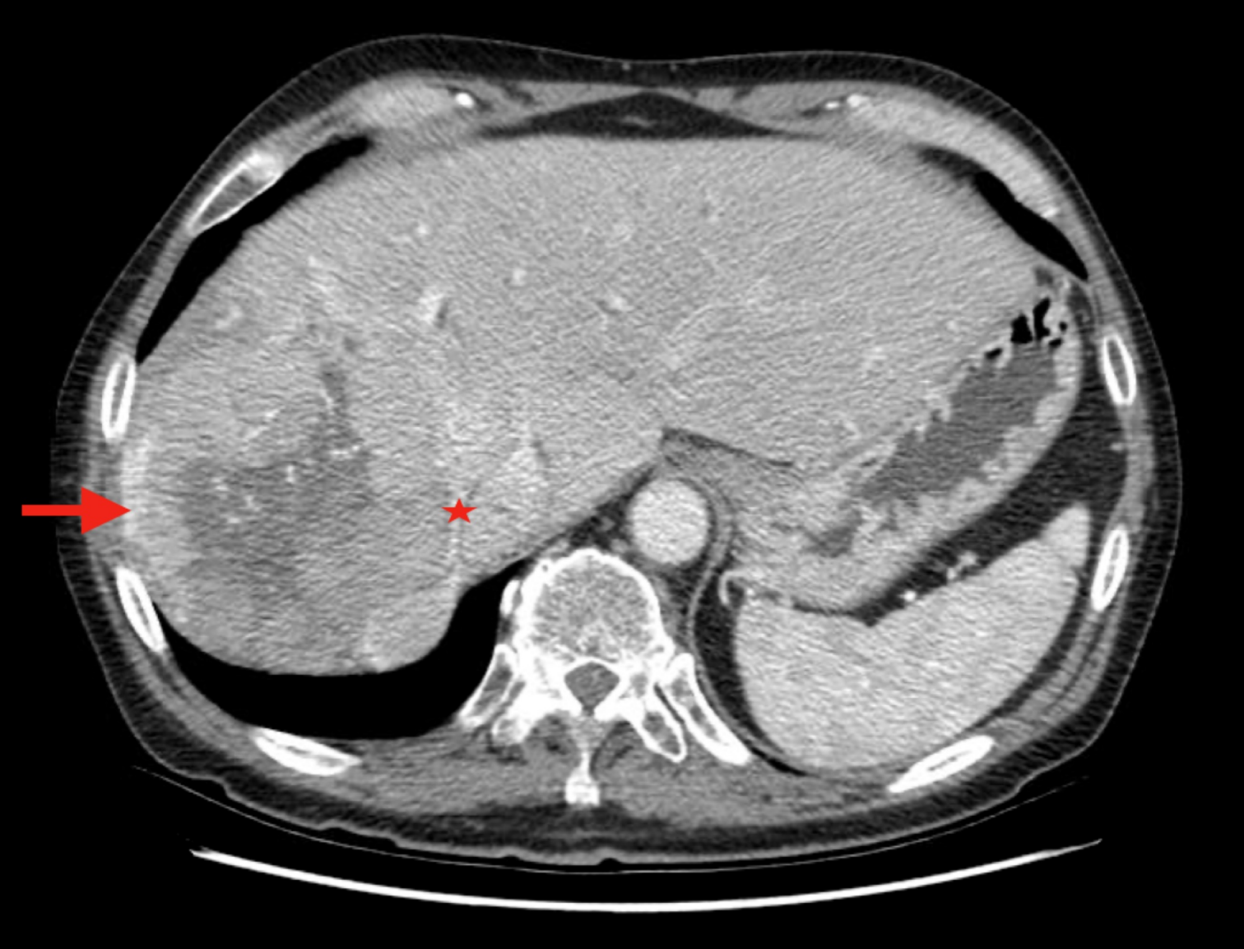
CT imaging 21 months after diagnosis Imaging continues to demonstrate a large right-sided heterogeneous mass (arrow), appearing larger than that in previous studies. The mass causes marked mass effect upon and narrowing of the inferior vena cava (star, medial to arrow).
